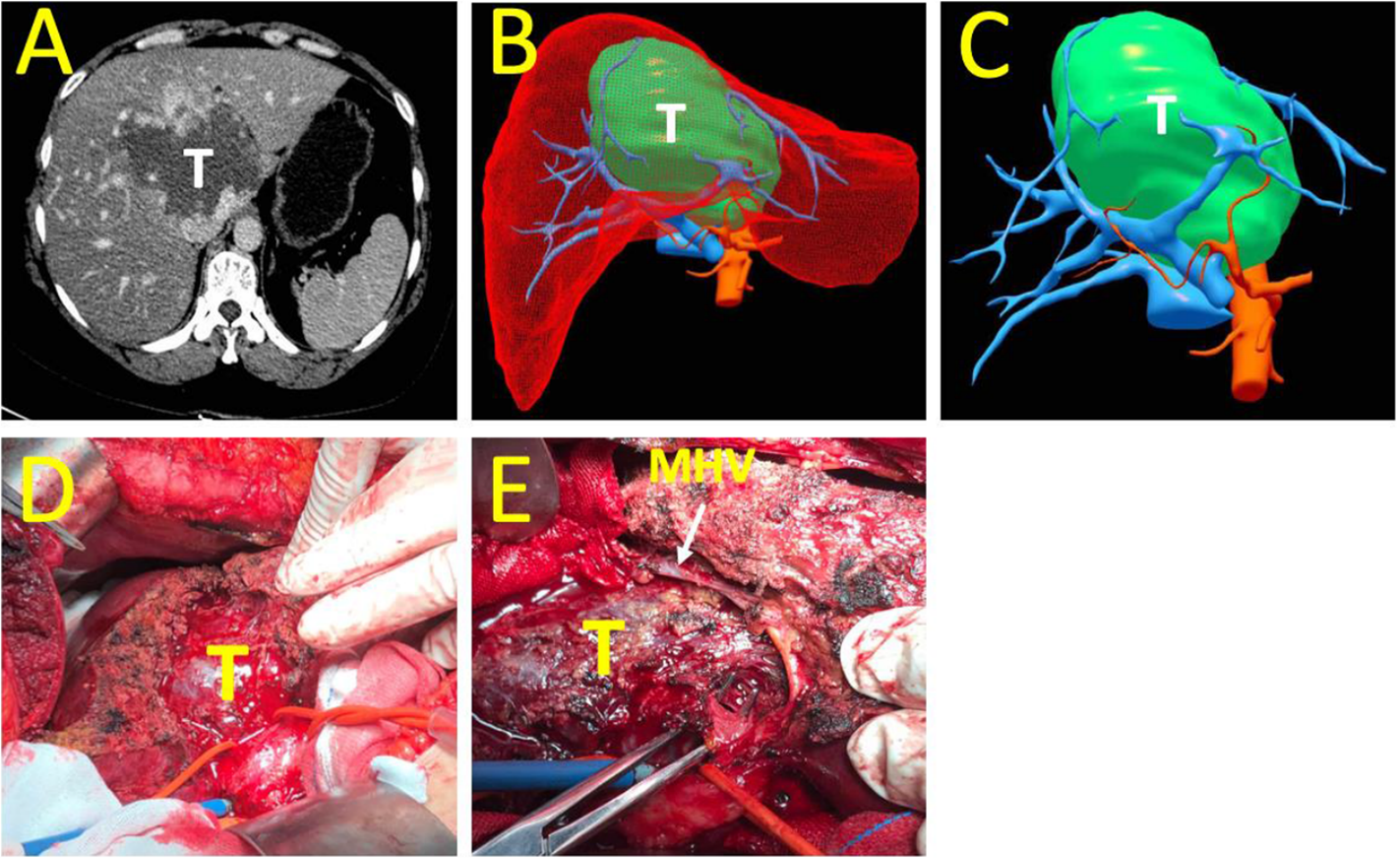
Isolated complete resection of caudate lobe (a CT scan showed a giant hemangioma in the whole caudate lobe. b and c Tumor vied by 3D reconstruction, T = tumor. d Tumor revealed through anterior transhepatic approach. e Tumor was beside the middle hepatic vein (MHV) and almost resected)
